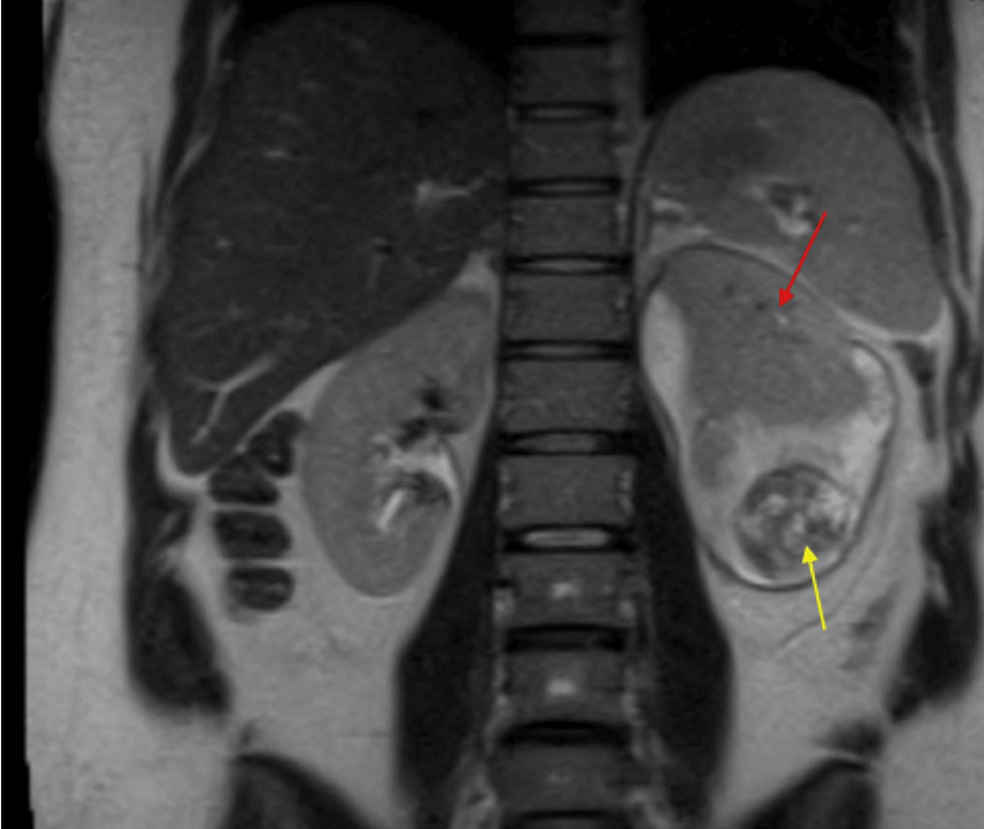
Coronal T2-weighted MRI abdomen showing left-sided inferior pole renal mass (yellow arrow) and subcapsular hematoma (red arrow)
